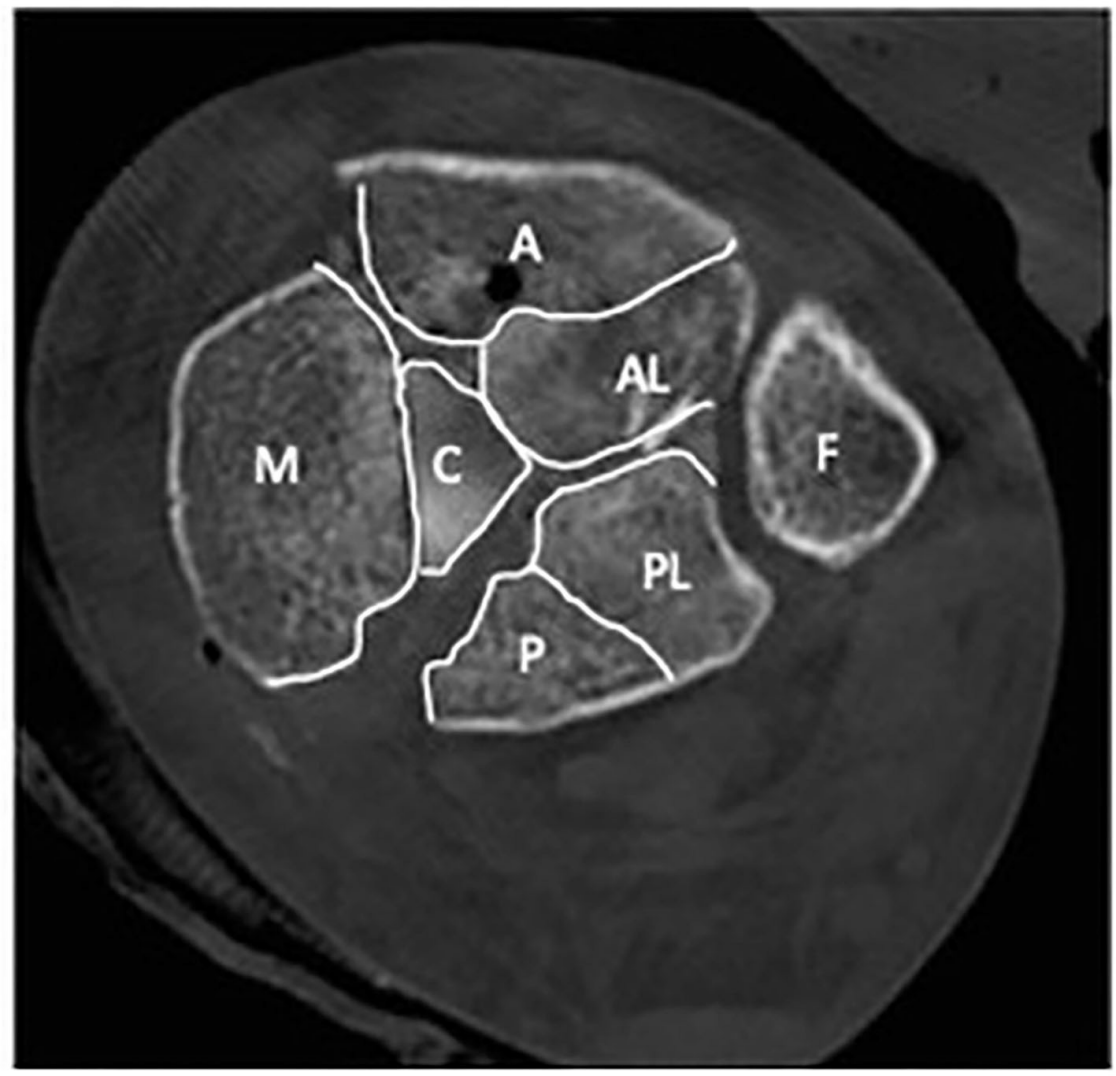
An axial CT of the distal tibia showing the typical six fracture fragments. A, anterior fragment; AL, anterolateral fragment; P, posterior fragment; PL, posterolateral fragment; C, central die-punch fragment; M, medial fragment; F, fibula.
